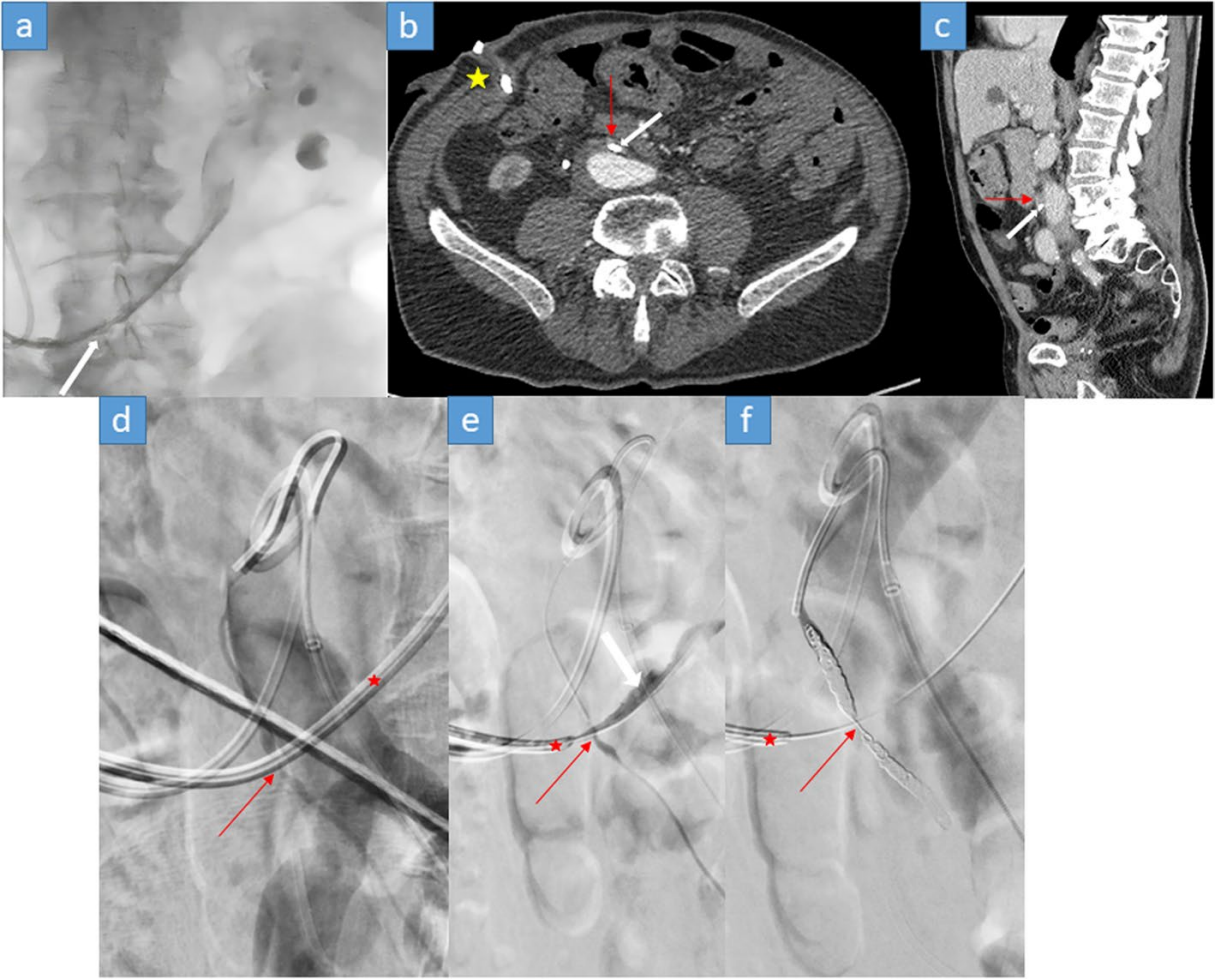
a R-UPG showing the flow from the ureter into a vascular structure (arrow). b and c A 2 months old CT scan showing the course of the left ureter (white arrow) crossing the midline between the aorta and the IMA (red arrow) after bilateral ureterocutaneostomy on the right side (star) with bilateral ureteral stents. d Overview DSA with an apparently normal IMA at the uretero-arterial crossing point (red arrow). Notice the lying left ureteral stent in place (star). e A selective DSA of the IMA using a microcatheter with direct visualization of contrast medium flowing into the ureter (white arrow) with a clear UVF at the uretero-arterial crossing point as direct visualization of the UVF (red arrow) after provocation by pulling the stent beyond the crossing point by the urologist (star). f Front door – back door coil embolization of the IMA with the starting point distal to the uretero-arterial crossing point (red arrow). Despite the stent remaining in a retracted position (star), complete elimination of the UVF through the coils was achieved
